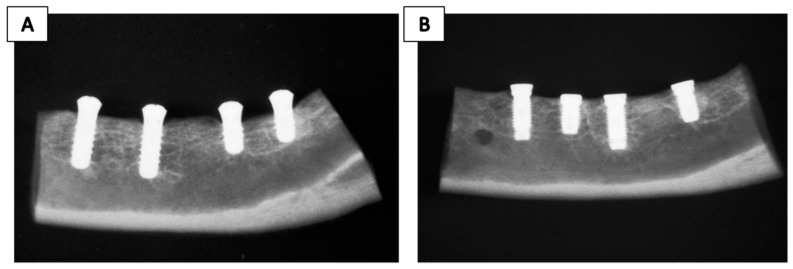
Radiograph taken after mandibular resection. Note that implants are embedded in the surrounding bone; no radiolucency was detected. (A) Bone segment with Brånemark implants, (B) bone segment with ITI-TPS^®^ commercial implants. Reproduced from Bernard et al. [71] with permission from John Wiley and Sons.
